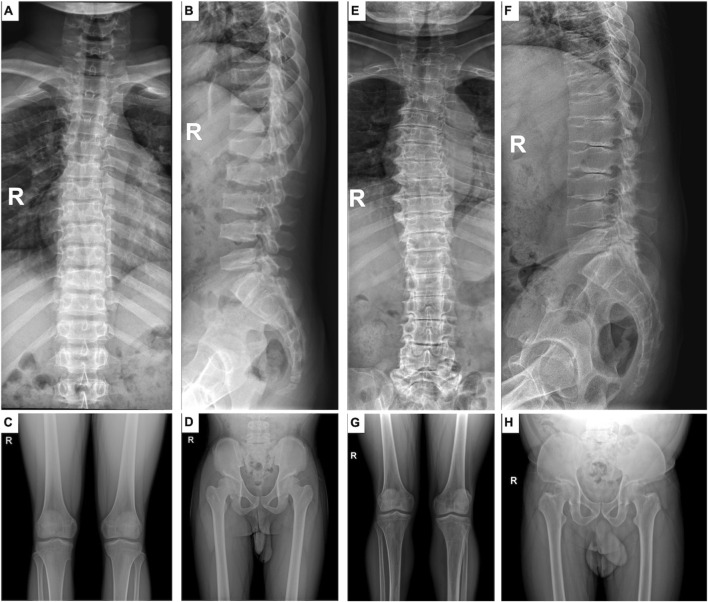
Features of radiograph with SEDL. (A–D) radiographs were taken at IV-1 16 years old. (E–H) radiographs were taken at III-13 32 years old. (A,E) Frontal view showing mild kyphoscoliosis. (B,F) Lateral view of the lumbosacral spine, revealing platyspondyly, hump-shaped central and posterior portions of vertebral bodies, and narrow disc spaces. (C,G) Frontal view of the knees, displaying mild flattened tibial plateaus. (D,H) Frontal view of the pelvis, indicating flat femoral heads and short femoral necks.
